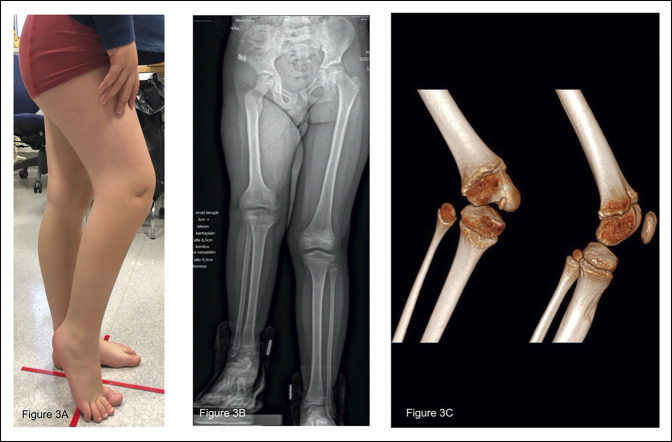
A, Photograph showing the clinical situation at the age of 7 years. B, Long lower limb radiograph at the age of 8 years showing 6.9-cm anatomical limb-length discrepancy. C, 3D computer tomography image showing the bony anatomy in the knee.
